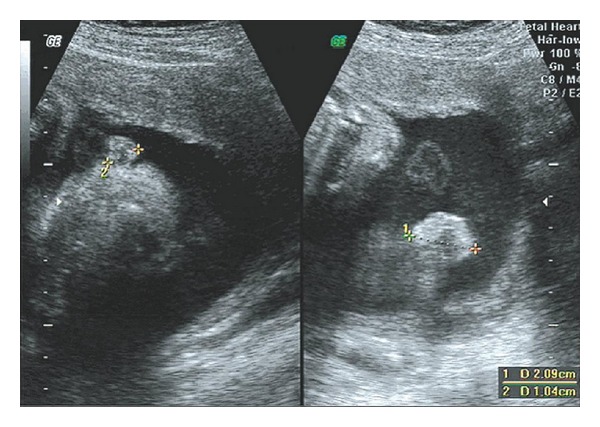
Sonogram of unilateral microtia on auricular parasagittal section.
